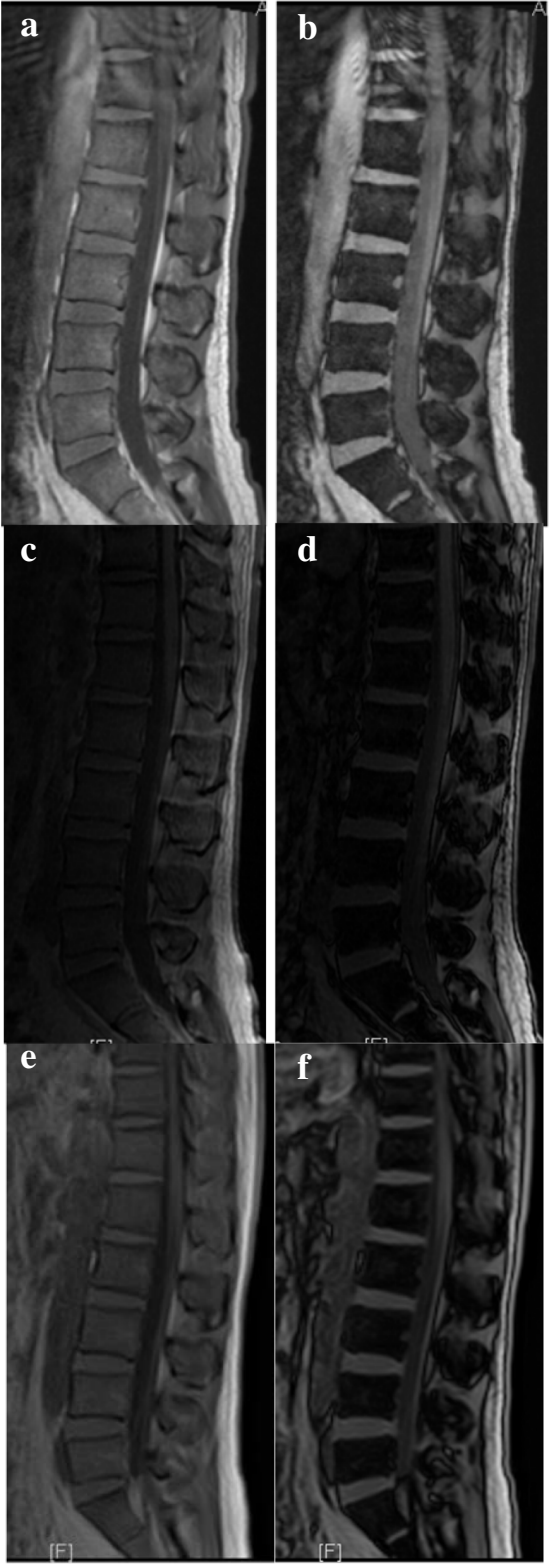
Representative images of chemical shift MR imaging in different MR systems. (a) in-phase image acquired at 3.0 T Siemens MR system; (b) out-of-phase image acquired at 3.0 T Siemens MR system; (c) in-phase image acquired at 1.5 T GE MR system; (d) out-of-phase image acquired at 1.5 T GE MR system; (e) in-phase image acquired at 1.5 T Toshiba MR system; (f) out-of-phase image acquired at 1.5 T Toshiba MR system
